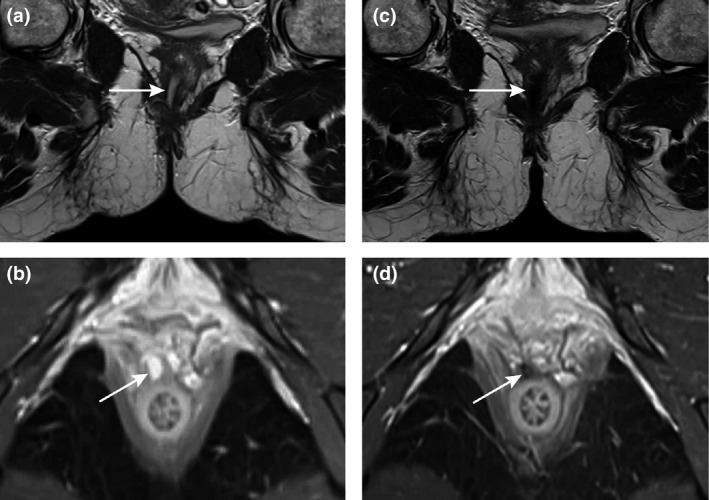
Completely fibrotic tract at 5‐months’ follow‐up. Coronal T2‐weighted images (a, c) and axial fat‐saturated T1‐weighted postcontrast images (b, d) at baseline (a, b) and at 5 months’ follow‐up (c, d) of a 34‐year‐old woman. MRI showed a fistula tract that changed from predominantly granulation tissue to predominantly fibrous 5 months after the ligation of the intersphincteric fistula tract procedure. The hyperintensity of the tract was pronounced at T1‐weighted postcontrast images at baseline (b) and absent at follow‐up (d). The patient showed clinical healing at the time of the follow‐up MRI.
